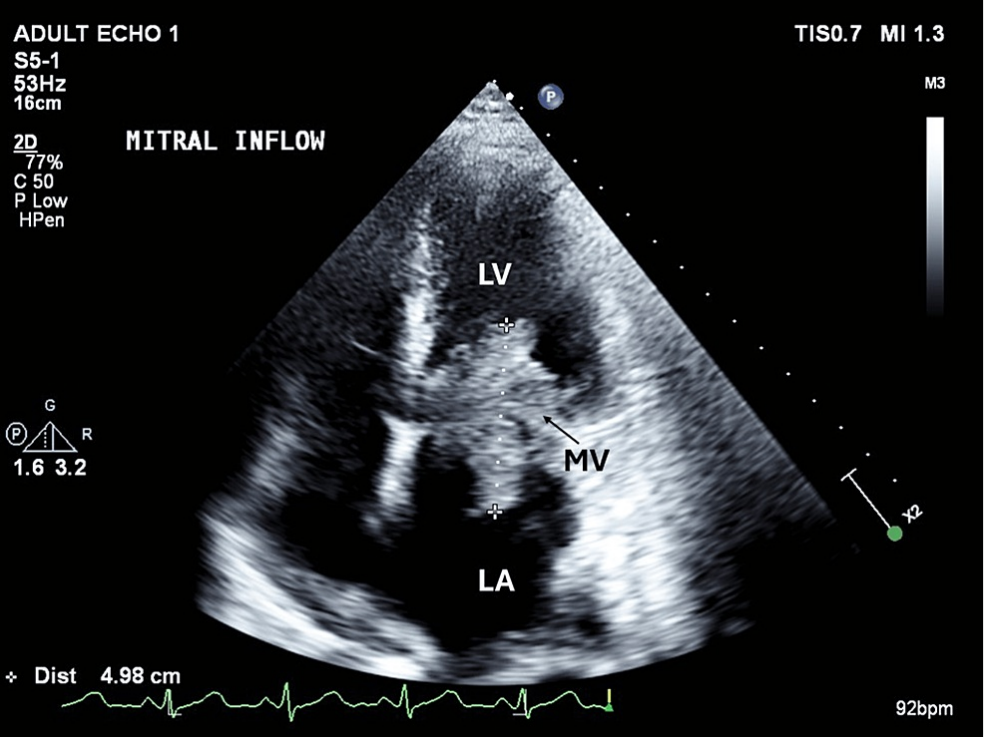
Transesophageal echocardiogram Showing around 5 cm mass attached to the left atrial (LA) wall and posterior leaflet mitral valve (MV) (arrow) with prolapse of segments of the mass into the left ventricle (LV) causing obstruction.
